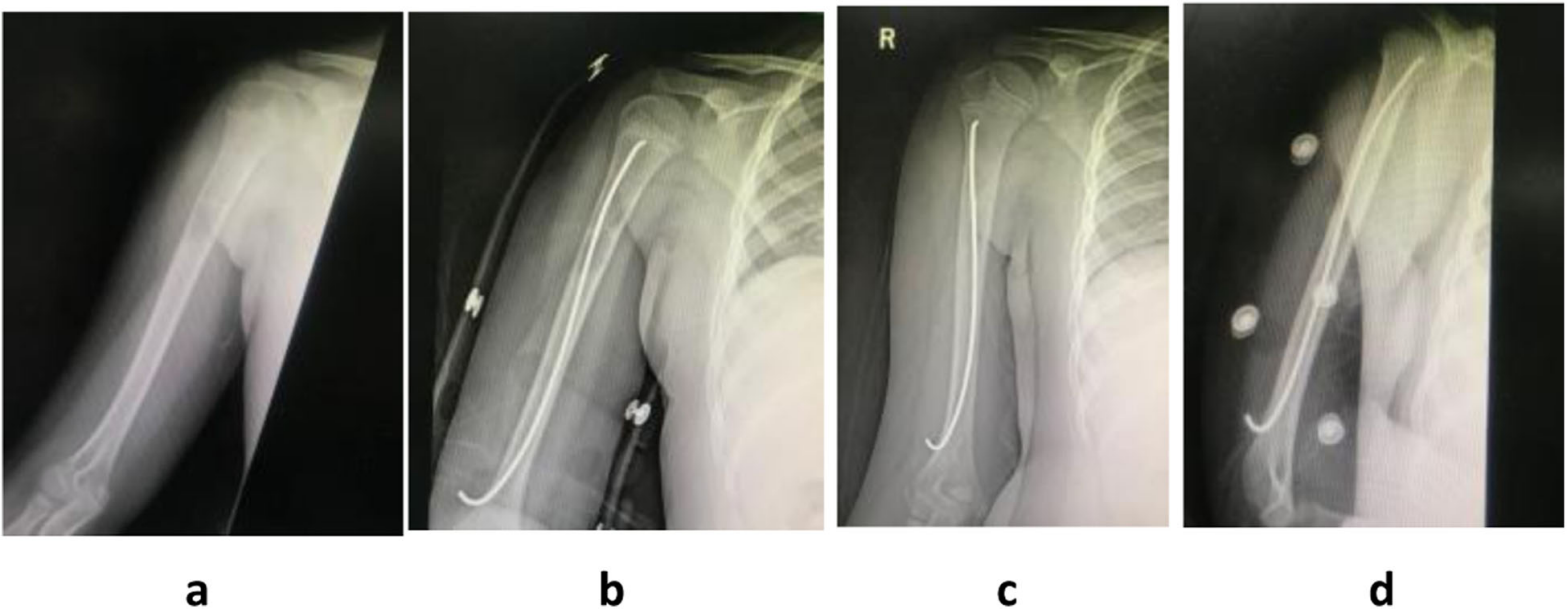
Male, eight years old, unicameral bone cyst localized in his right proximal humerus. 3a X-ray before the implantation of ESINs. 3b X-ray image after the implantation of ESINs. The ESINs runs through the cyst. 3c X-ray image at three months after the operation and there is new osteotylus in the cyst. 3d X-ray image at six months after the operation showed more osteotylus formed in the cyst. The bone cyst was healed
